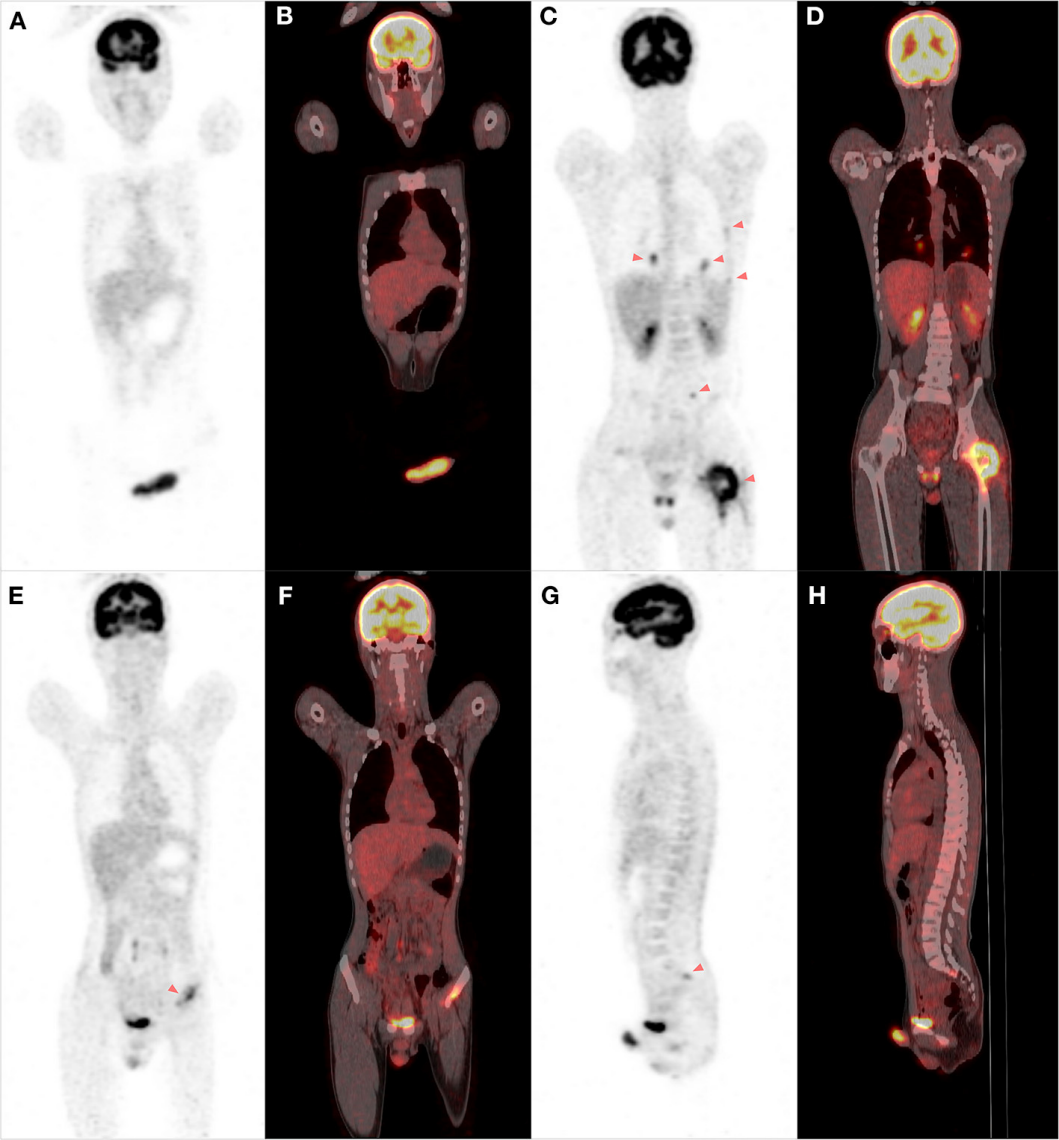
The FDG PET/CT scan of the whole body. A PET-CT scan showed increased fluorodeoxyglucose uptake in the lesions of the penis and *corpus cavernosum*
